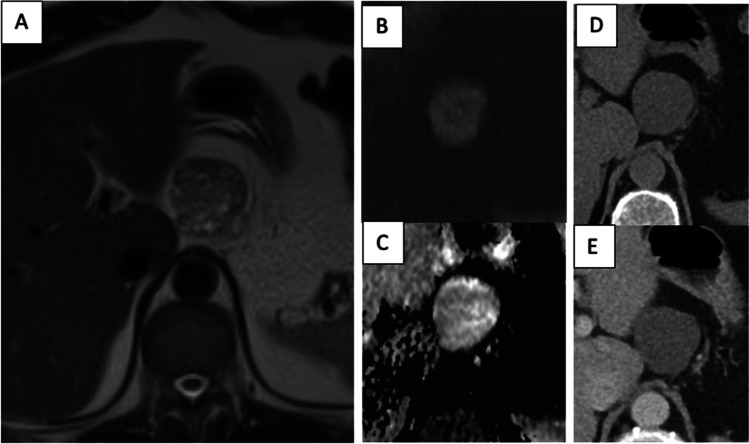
A 48-year-old patient with a 4,5 cm size lymphoepithelial cyst (LEC) in the pancreatic body with an exophytic location. Axial T2-weighted MR image (a) shows diffuse subsolid morphology of the lesion. Axial DWI MR image (b) and axial ADC MR image (c) show no restricted diffusion. Axial CT image in native phase (d) shows an isodense CT attenuation of the lesion. Axial CT image in venous phase (e) shows no significant enhancement of the lesion
